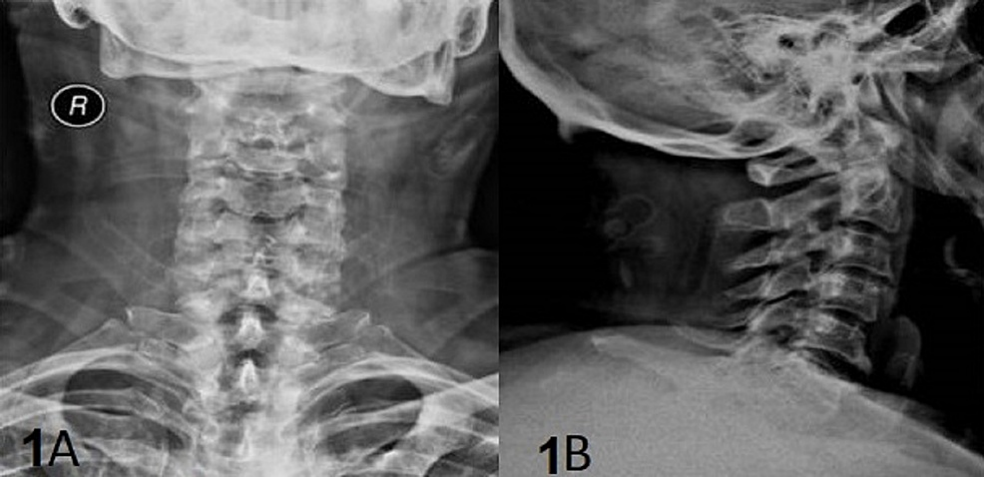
X-rays of the cervical spine A 60-year-old man patient with cervical spine's trauma who presented with severe pain of the cervical spine, headache, and numbness of his arms: (A) X-rays of the cervical spine (anteroposterior view) didn't show any pathology; (B) X-rays of the cervical spine (lateral view) didn't show any pathology. Both X-rays are performed with an orthopedic collar.
